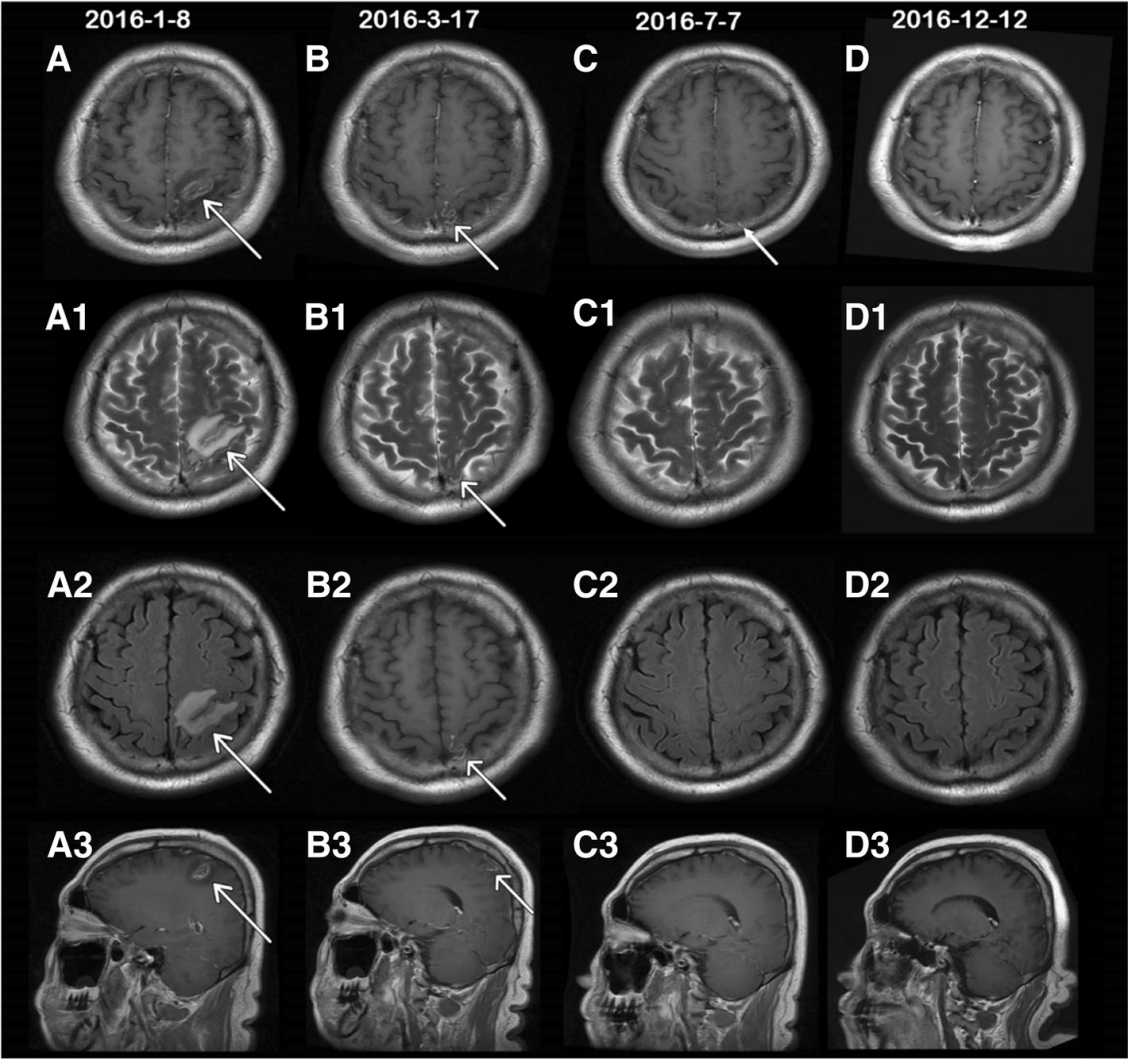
head MRI scan during three hospital stays and 1 year follow-up. a-d T1WI + FLAIR+contrast enhancement MRI on 4 occasions; A1-D1 T2WI; A2-D2 T2WI + FLAIR; A3-D3 sagittal T1WI + FLAIR+contrast enhancement. A. A3 shows MRI scan during the first hospital stay in January 2016. T1WI with arrowhead shows horseshoe lesion with short signal and irregular enhancement. Both T2WI and T2WI + FLAIR have long signals. B.B3 shows MRI scan in March 2016 showing veil-like long T1 and long T2 signals in the parietal lobe with obvious enhancement. This is considered to be the dead parasite bodies after deworming treatment. Tunnel sign caused by parasite migration is shown in B3 with arrow. C.C3 shows MRI scan in July 2016. The arrow indicates few spot enhancements in T1 + C with minimum lesion present. D.D4 shows MRI scan in the first-year follow-up in December 2016. No obvious abnormal lesions were seen
